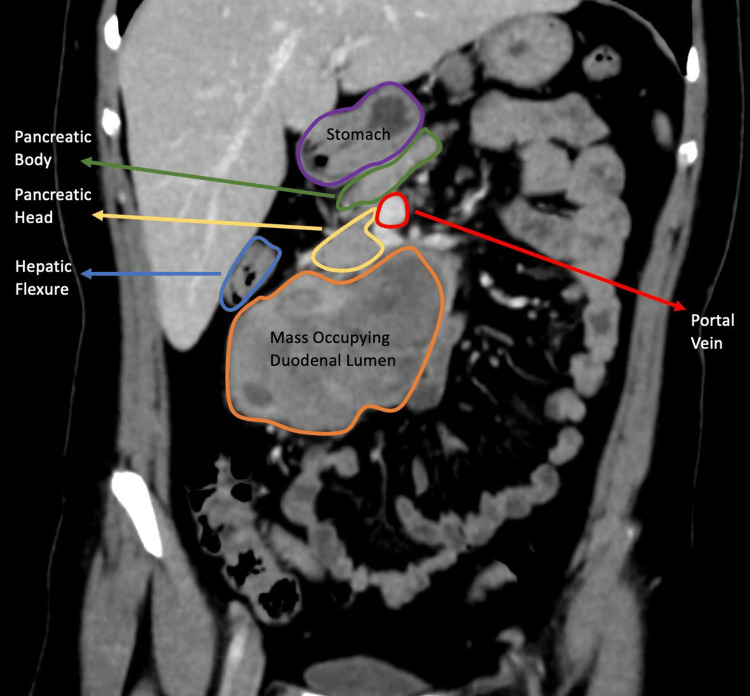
Contrast-enhanced coronal CT image of an intraluminal duodenal mass A large heterogeneously enhancing intraluminal duodenal mass is seen. Distally, the lesion extends along the lumen and forms an acute angle with the duodenal wall.
